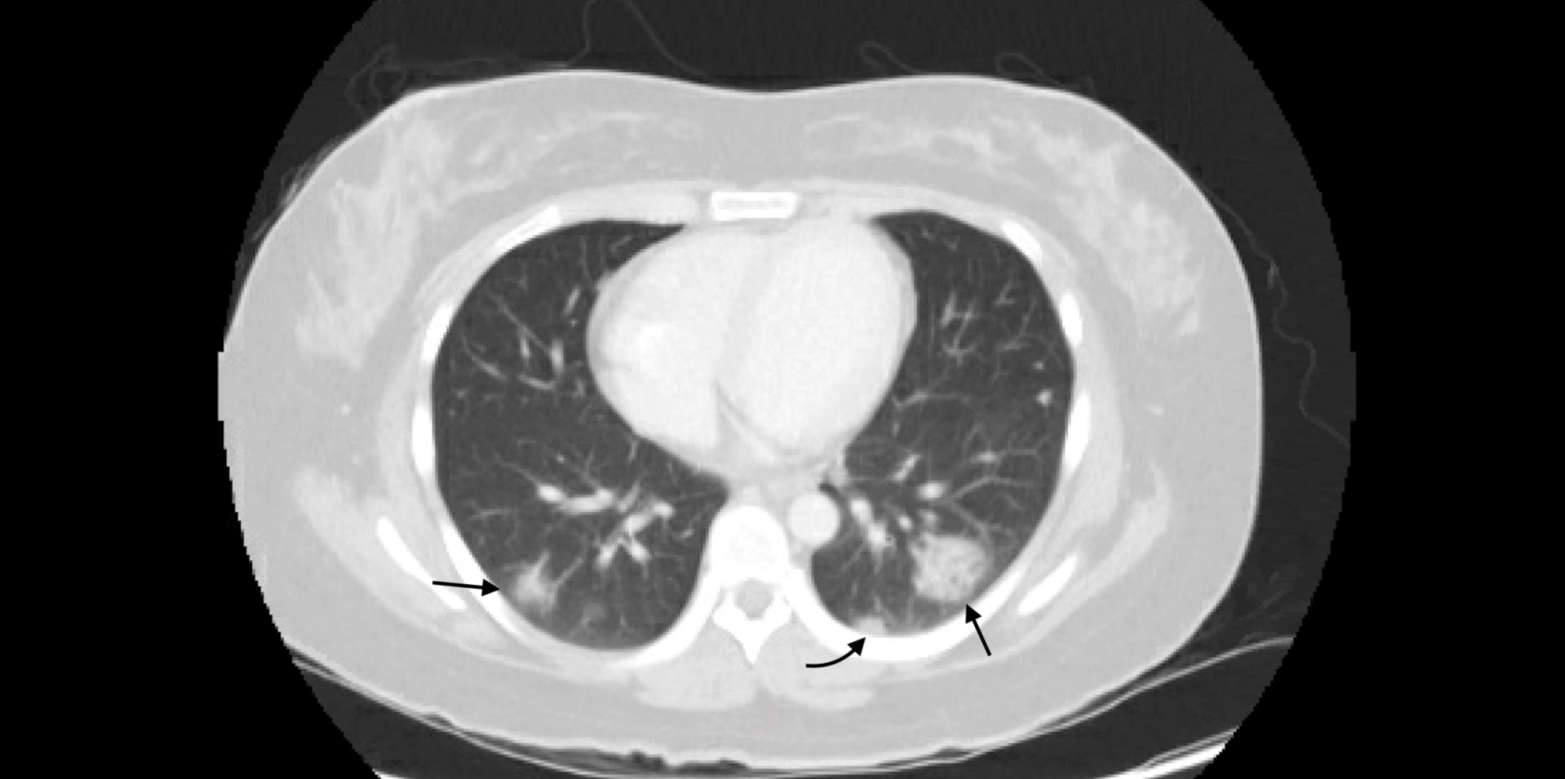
CT of the chest with contrast revealing heterogeneous cavitary opacities at the bilateral lower lobes
